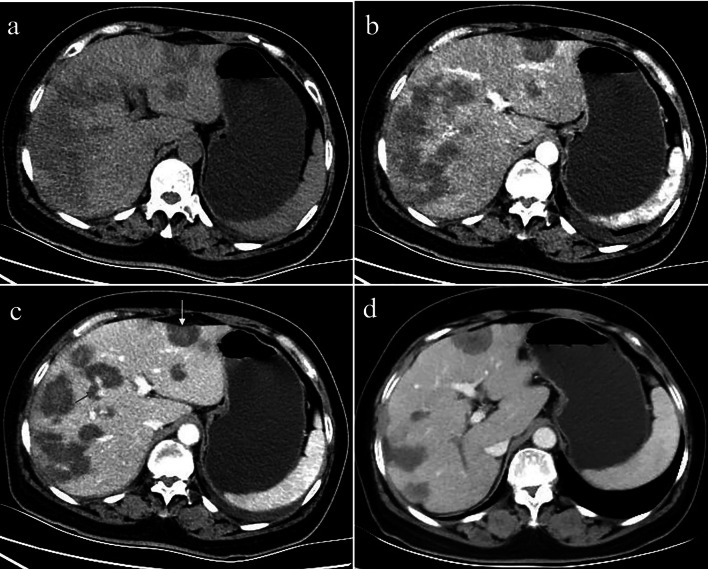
A 52-year-old female with diffuse nodular type HEH. Axial unenhanced CT images (a) show diffuse coalescing hypoattenuating lesions. Arterial phase CT images (b) show slight enhancement, and some nodules confluent to strip‑like or snowmelt are located subcapsularly in the liver. In the portal phase (c), the lesions are not progressively enhanced, a small branch of the portal vein is seen passing through the middle of the lesions (red arrow), and capsular retraction (yellow arrow). On follow-up CT performed 10 months after radiofrequency ablation, axial portal vein phase CT image (d) shows lesion reduction
